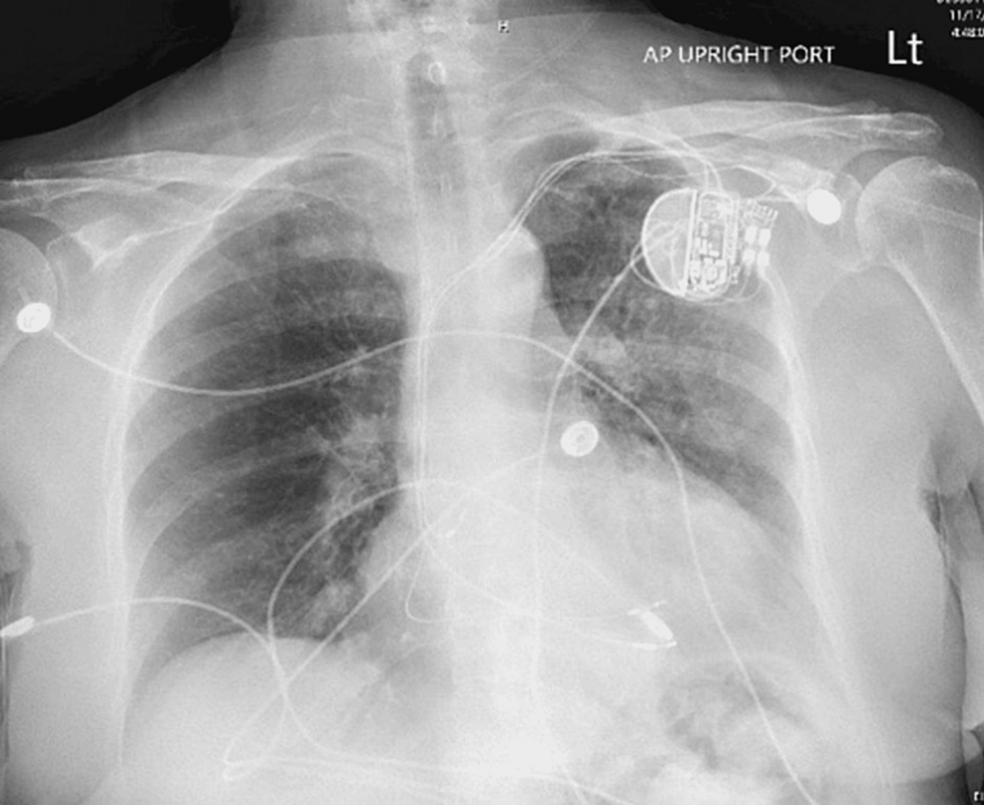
Chest X-ray shows developing pneumonia on the left.
